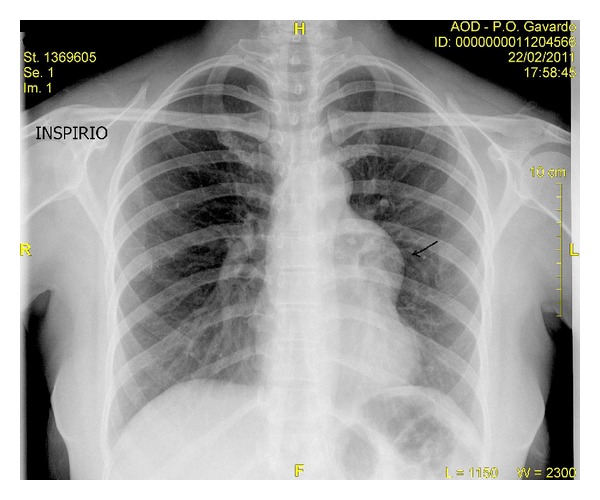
Chest X-ray in P-A: opacity mass of about 4.5 cm skull-caudal extension in left lateral anterior mediastinum.
